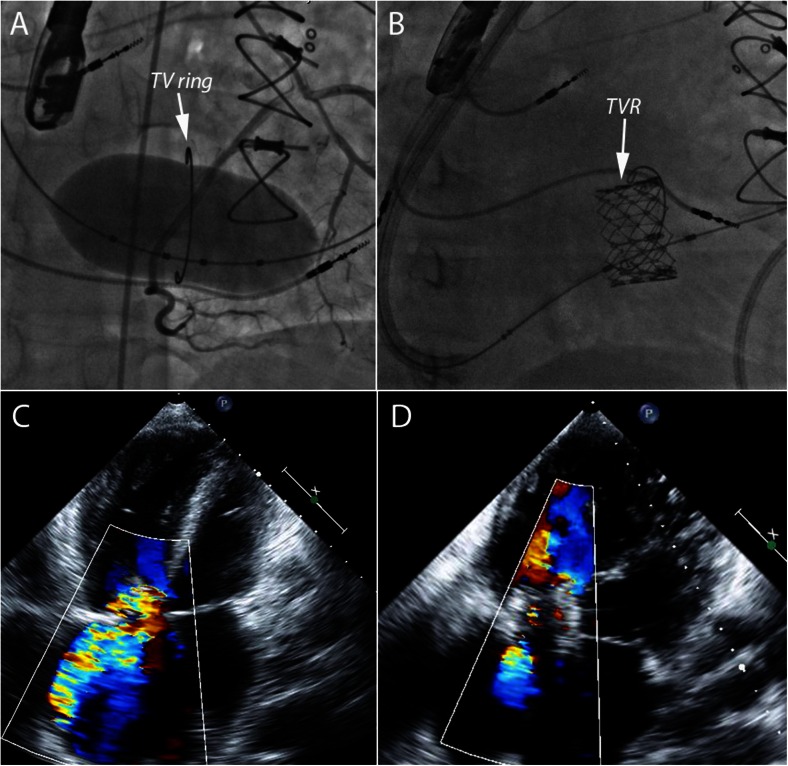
a Right anterior oblique (RAO) angiographic view of an inflated 30-mm balloon across a previously surgically placed incomplete tricuspid valve ring (*white arrow*). Simultaneous selective right coronary artery injection shows no evidence of compression. b Sapien transcatheter valve replacement (TVR) within the ring. c Transthoracic echocardiographic image (apical four-chamber view) with color Doppler flow showing severe tricuspid regurgitation across the native tricuspid valve. d Transthoracic echocardiographic image (apical four-chamber view) with color Doppler flow showing mild regurgitation across the Sapien valve
